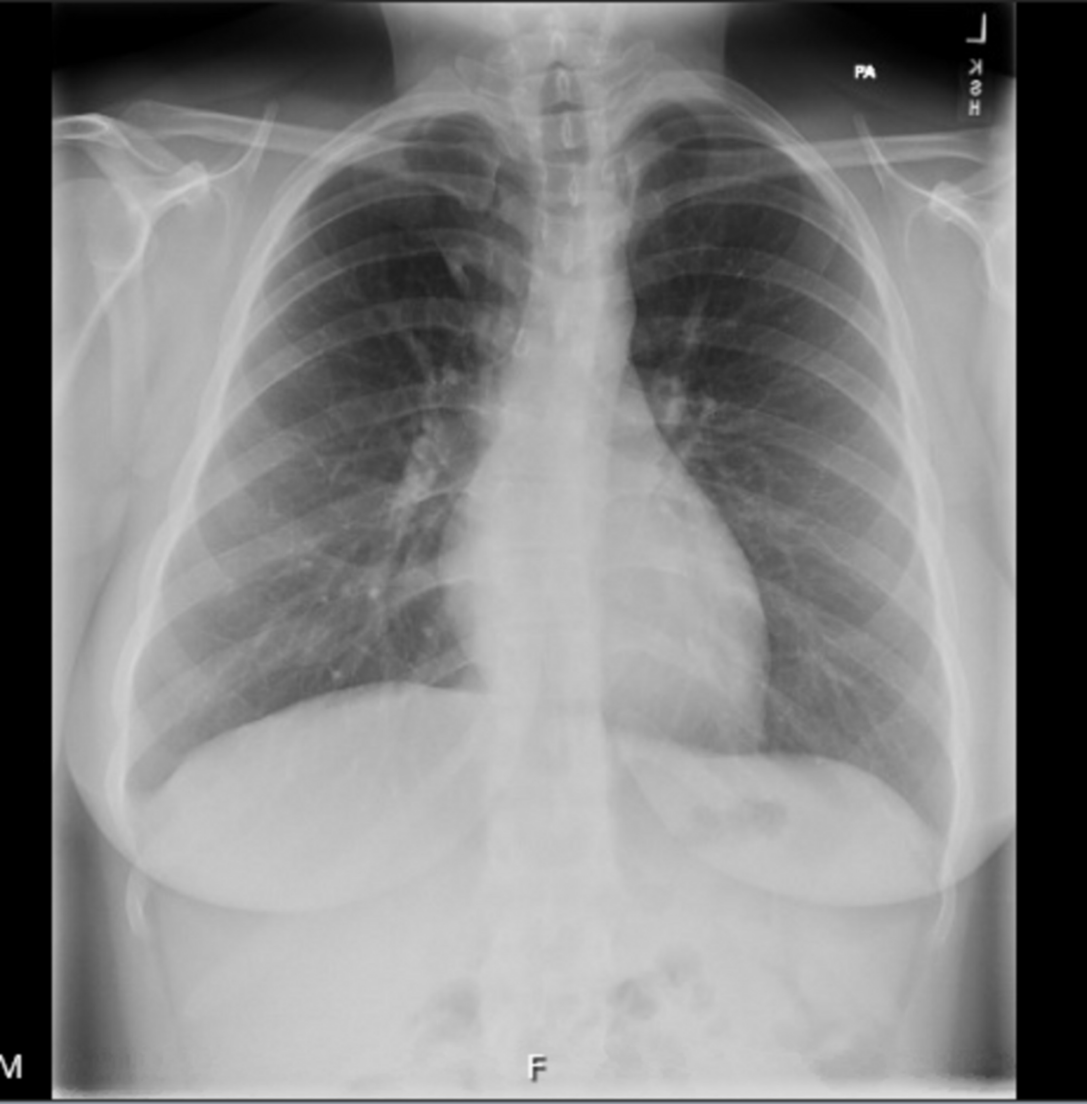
Normal chest X-ray of the patient
